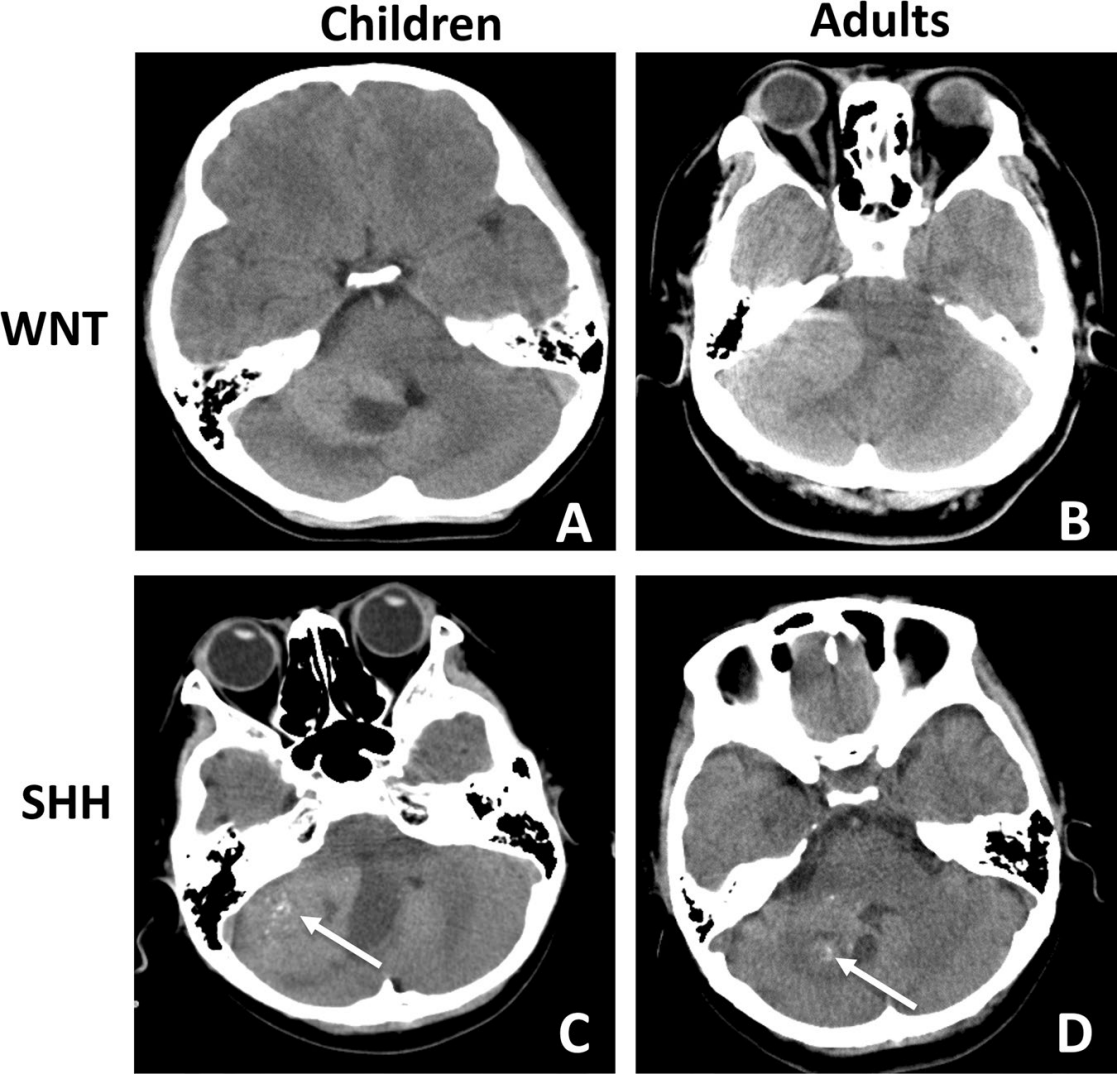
Exemplary CT scan (axial) of cerebellopontine angle medulloblastoma (MB). (A,B) Pediatric and adult CPA WNT-MBs are shown. (C,D) Pediatric and adult CPA SHH-MBs with mineralization (white arrow) are shown. No obvious bone erosion or dilation of the IAC is found in any of the cases.
